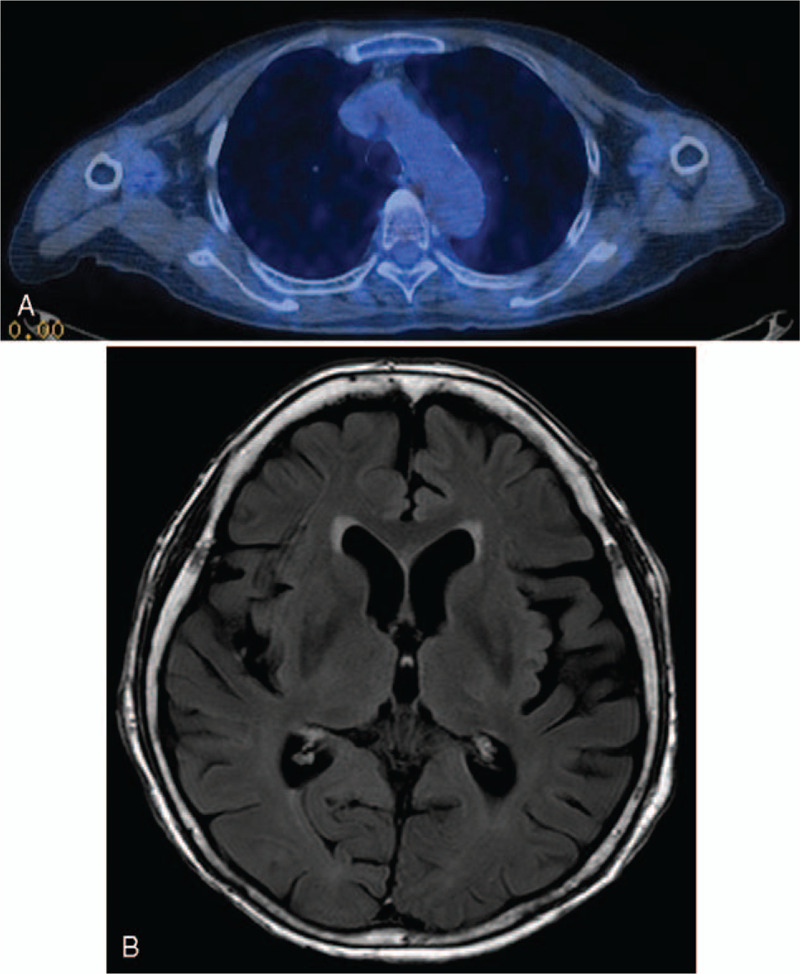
One year after treatment, a follow-up positron emission tomography-computed tomography scan (A) and brain magnetic resonance image (B) on March 18, 2015. The metastatic bone and brain lesions had disappeared.
